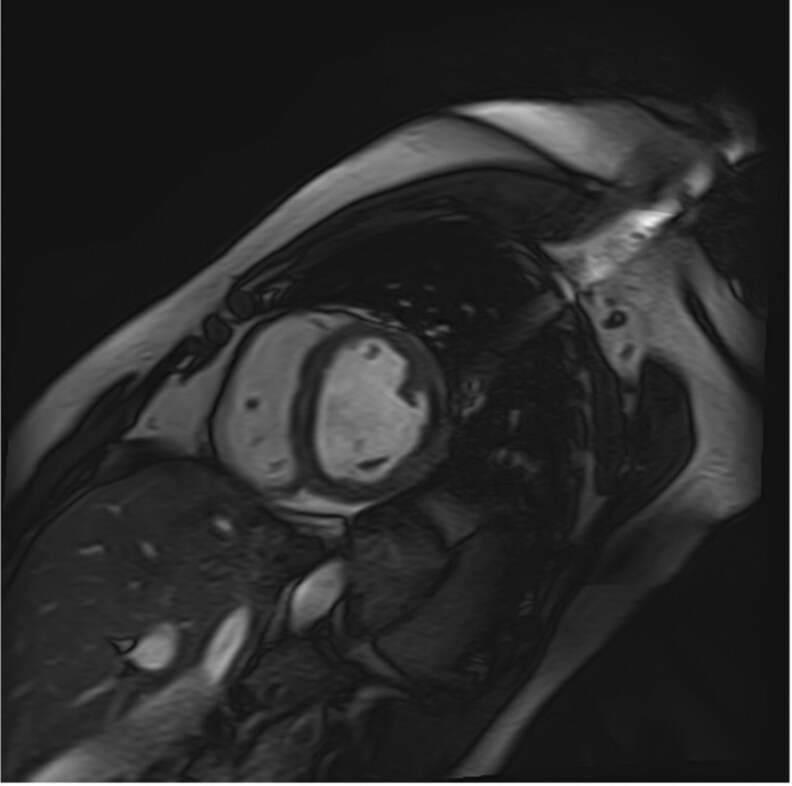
A follow-up cardiac MRI displayed a complete disappearance of the mural thrombus.
